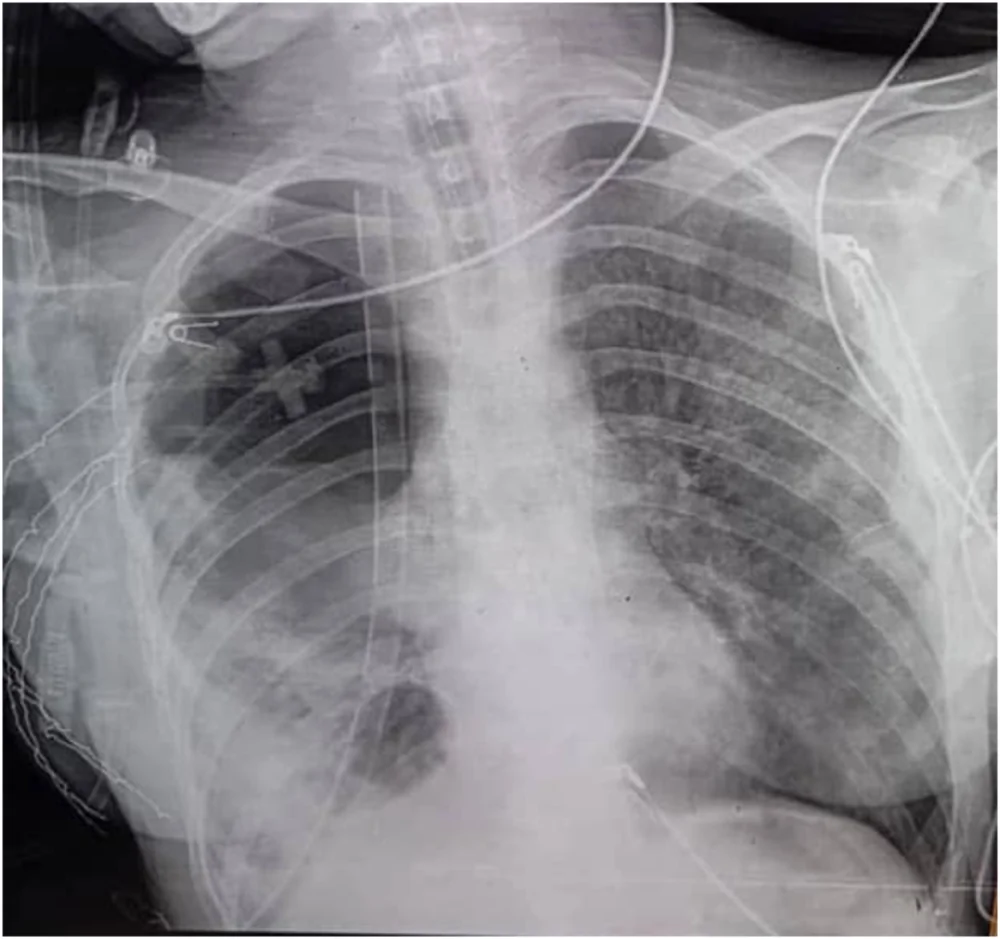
Portable anteroposterior chest radiograph obtained approximately 3 hours after surgery shows a right pleural chest tube, persistent right pleural air with incomplete re-expansion of the right lung, and bilateral diffuse alveolar–interstitial pulmonary opacities representing early postoperative lung injury.
